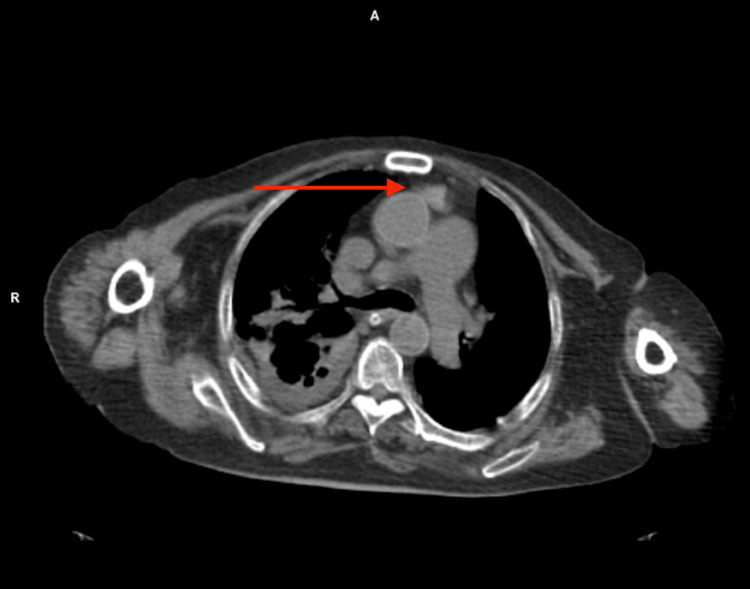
Thorax CT section. Arrow: a lobulated mediastinal image with features suggestive of thymoma.
